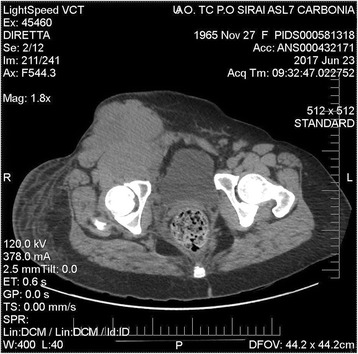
Case 2: axial computed tomography scan showing the lymph node mass (14 × 15 cm) extending to the origin of the right thigh
